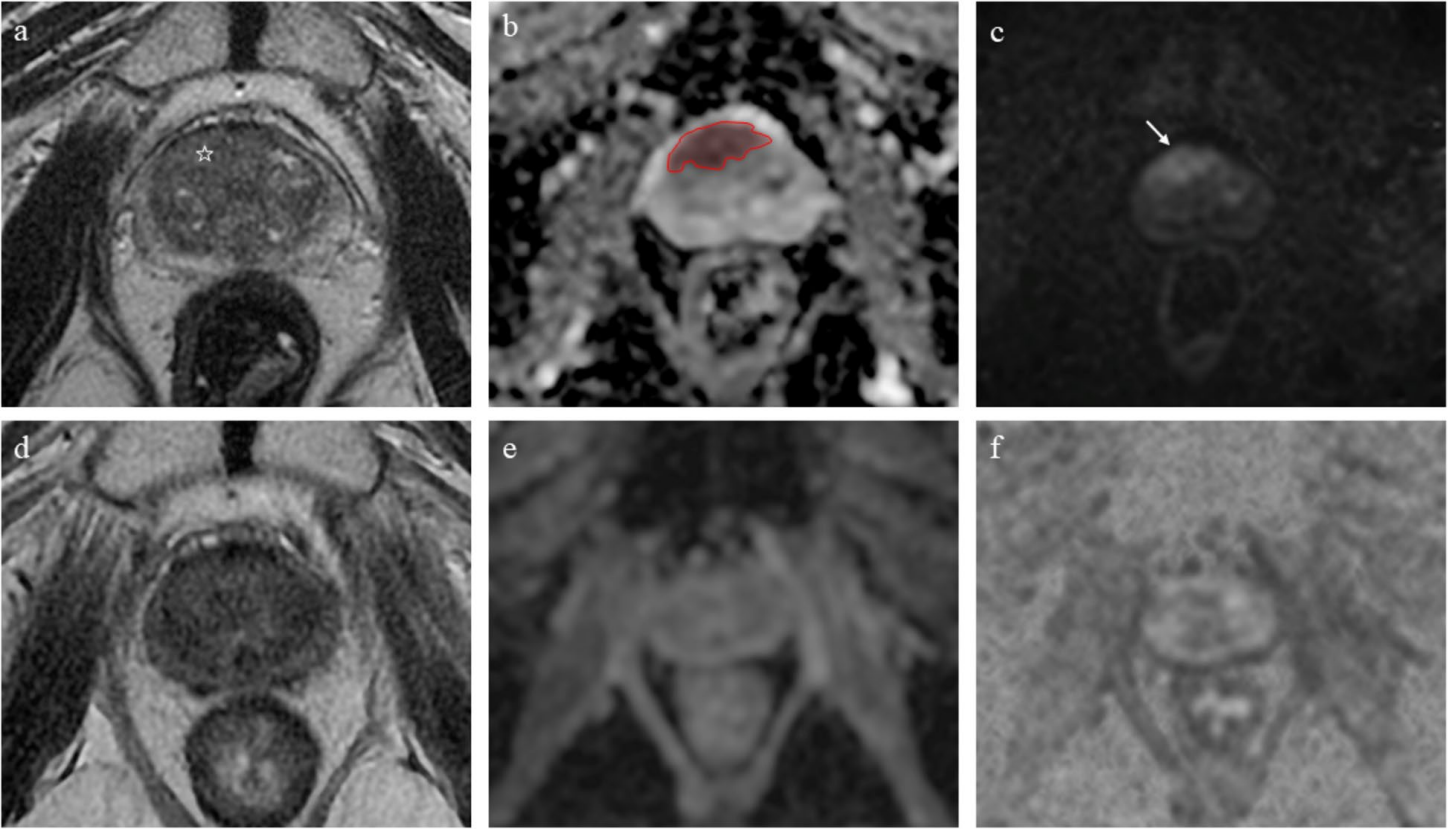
Sixty-nine-years old patient treated with external beam radiotherapy after MRI targeted biopsy revealed 3+4 Gleason score prostate cancer. Images from pre-biopsy (a−c) as well as post-treatment (d−f) MRI scans are presented. The index lesion (PI-RADS 5) can be appreciated on the T2-weighted images (a, lesion epicentre marked with a white star) as a homogeneous, moderately hypointense area with obscured margins in the right anterior transition zone. Corresponding marked and focal hypointensity on ADC map (b, red region of interest) and hyperintensity on high *b* value DWI (c, white arrow) are present. On the corresponding post-treatment sequences (d−f), no abnormalities can be detected, suggesting a good treatment response. *DWI* Diffusion-weighted imaging, *MRI* Magnetic resonance imaging, *PI-RADS* Prostate Imaging-Reporting and Data System
